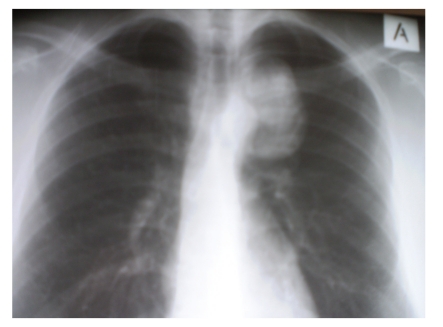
The posteroanterior chest radiograph shows a well-defined radiopaque lesion.
